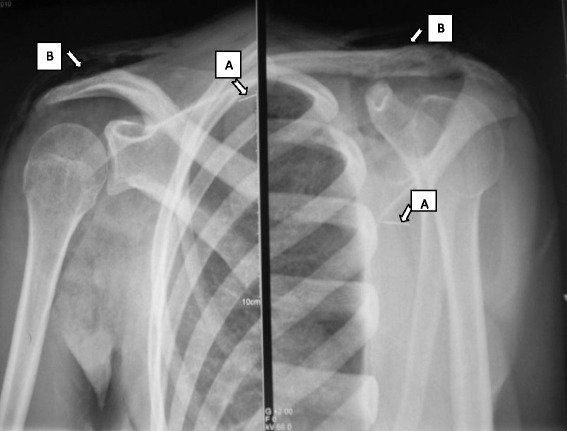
Front and three quarters shoulder radiography showing the presence of the needle in the subscapularis (arrow A) and subcutaneous emphysema in the shoulder (arrow B)
